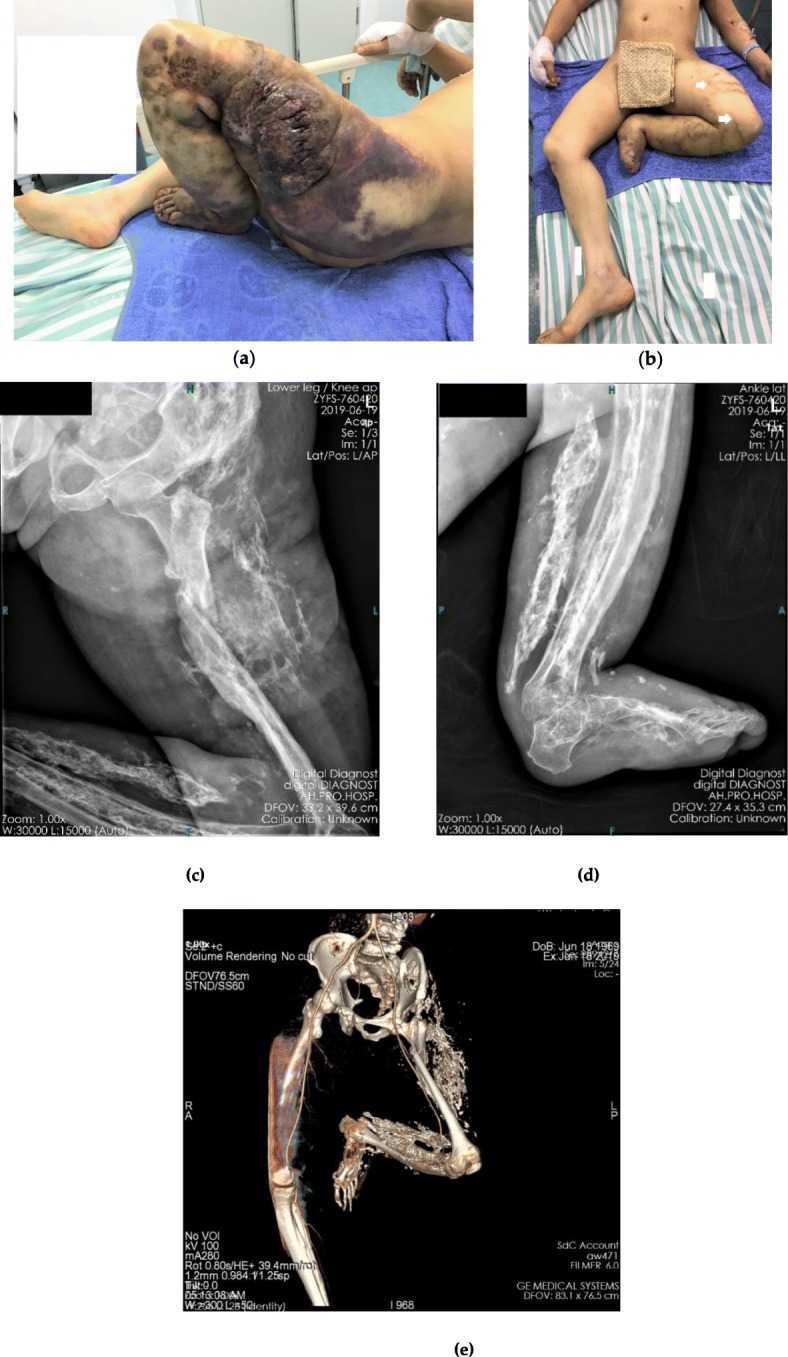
Photographs and images of the injury. a Initial presentation of hemangioma, a 15-cm stitched wound can be seen on the hemangioma; b Contracture of the leg, the white arrows show superficial varicose veins; c-d Radiograph showing femoral fracture and extensive high-density shadows distributed at the flexor muscle side of left lower extremity; e Three-dimensional computed tomography (3DCT). Vascular reconstruction shows coherence and integrity of the main arteries of the lower extremities
